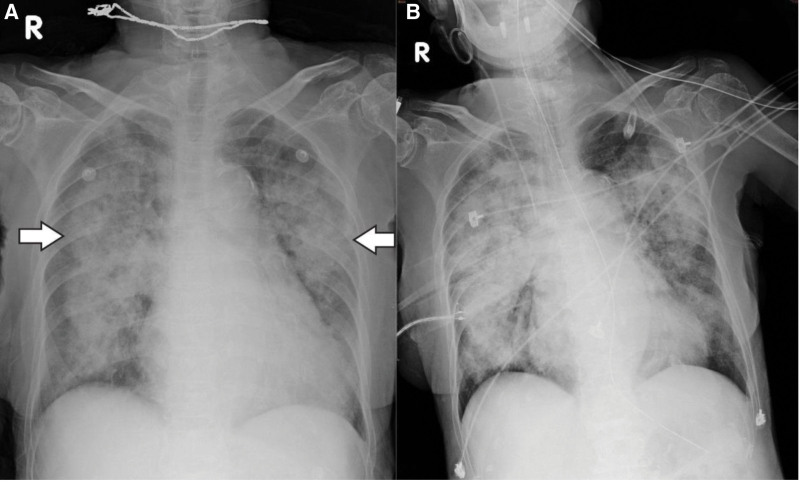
Chest plain film in the emergency department, revealing large bilateral consolidations suspicious for pulmonary edema or pneumonia.
